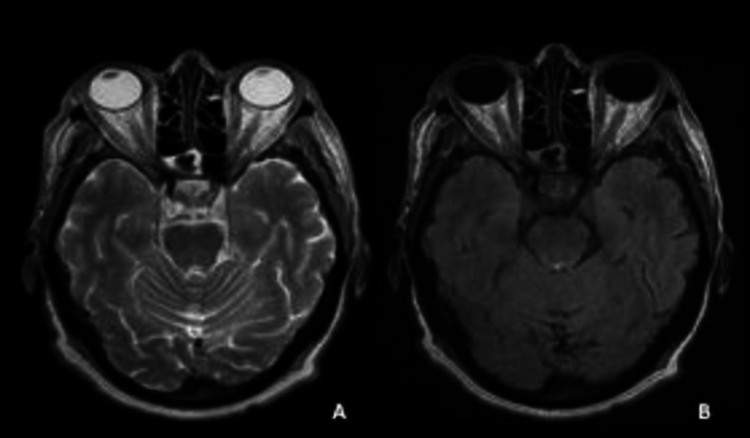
Orbital magnetic resonance imaging (1.5 Tesla) reveals absence of signal abnormalities on the optic nerve A) Axial T2 view; B) Axial T2 fluid-attenuated inversion recovery (FLAIR) view
